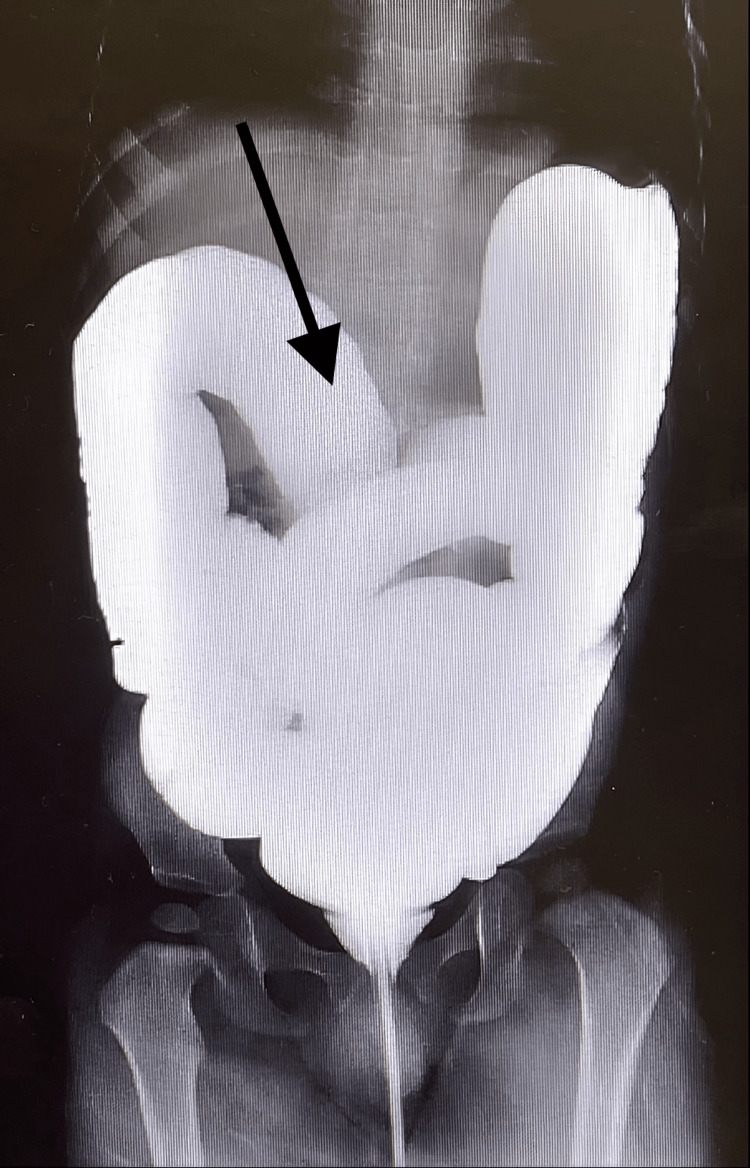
Day 2 X-ray gastrografin enema image showing the reduced obstruction
